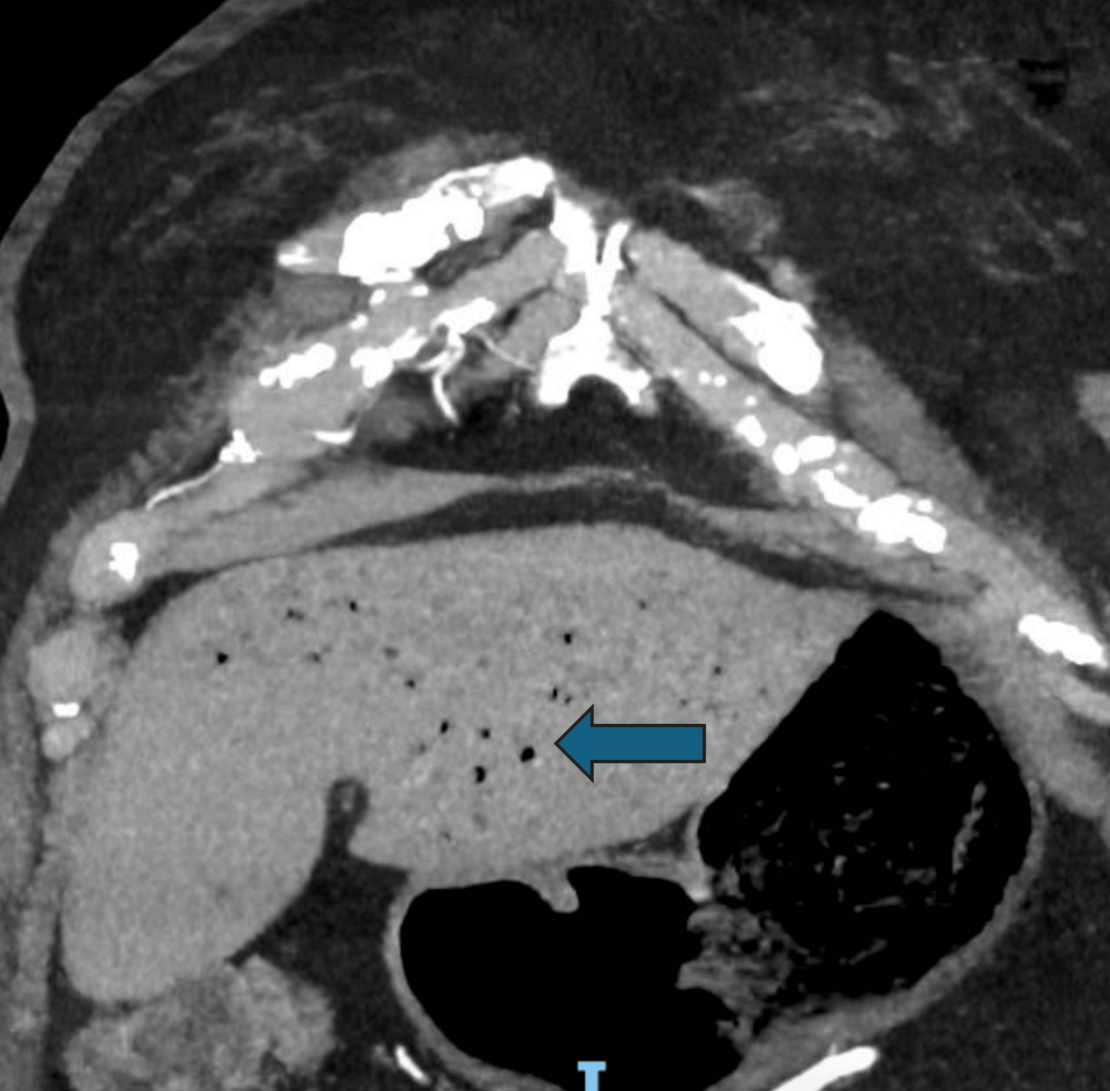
CT angiography showing portal venous gas (arrow)
